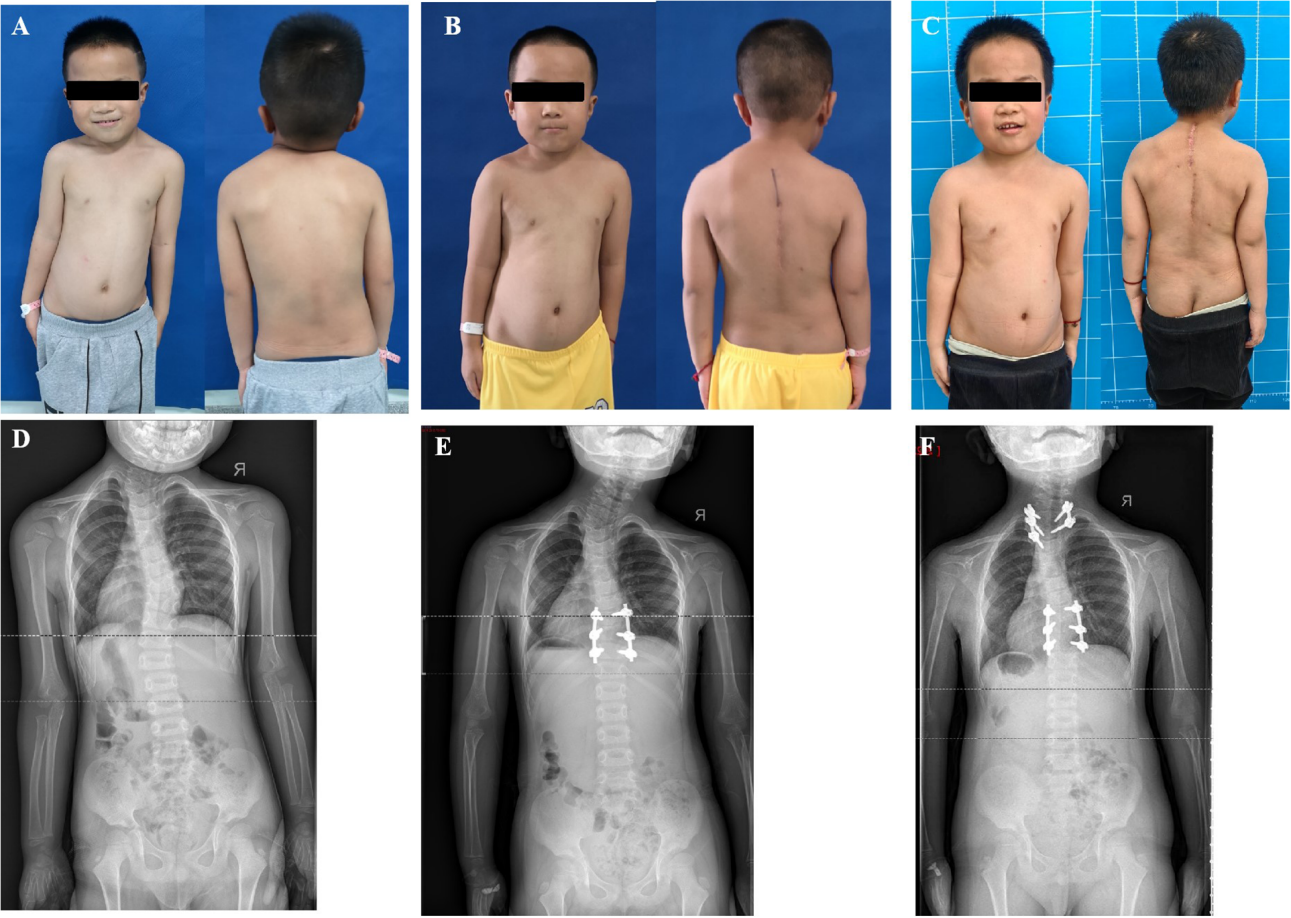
A 6 years 3 months old boy presented shoulder imbalance and facial asymmetry. Radiographs indicated that T2 and T10 were both hemivertebra and they compensated each other before the operation (A,D). He underwent a staged operation. The T10 HV was removed at the first surgery and he gained good shoulder balance and worsening torticollis due to the lack of compensatory T10 HV 3 months post the first operation (B,E). T2 HV was resected a second time, and his facial cosmesis and torticollis improved significantly 3 months post the second surgery (C,F).
